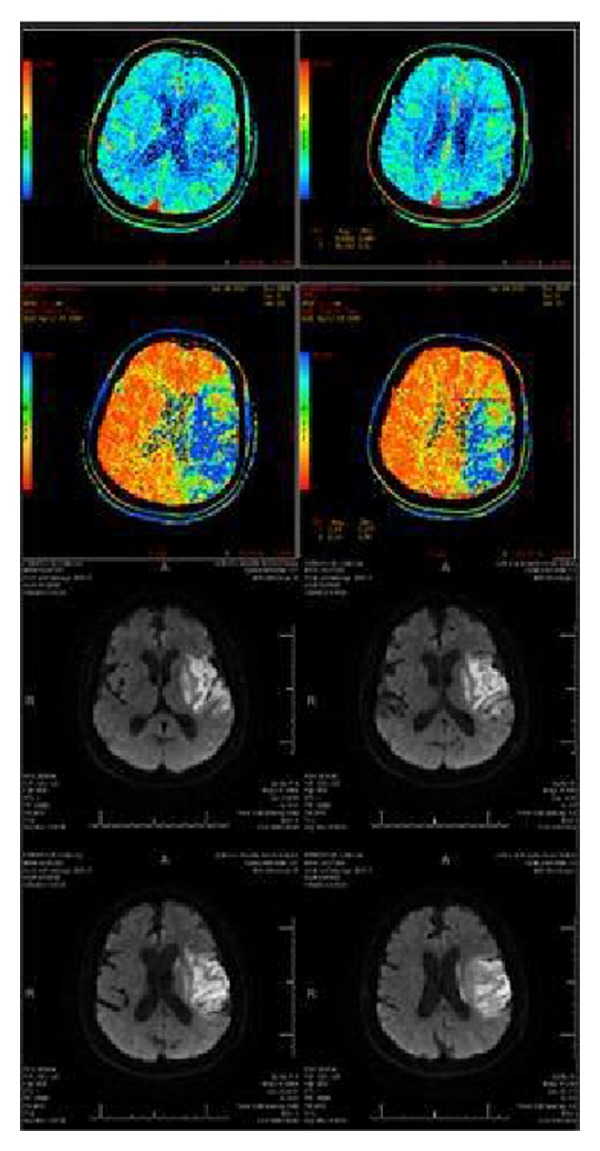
Top shows CT head perfusion study with mismatch in mean transit time and blood volume. Bottom shows smaller final completed stroke area in DWI sequence of MRI. Both used to calculate percentage area salvage.
